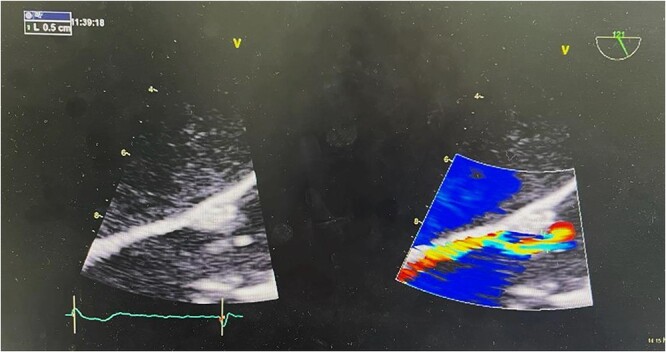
Transesophageal echocardiography showed severe aortic regurgitation.
